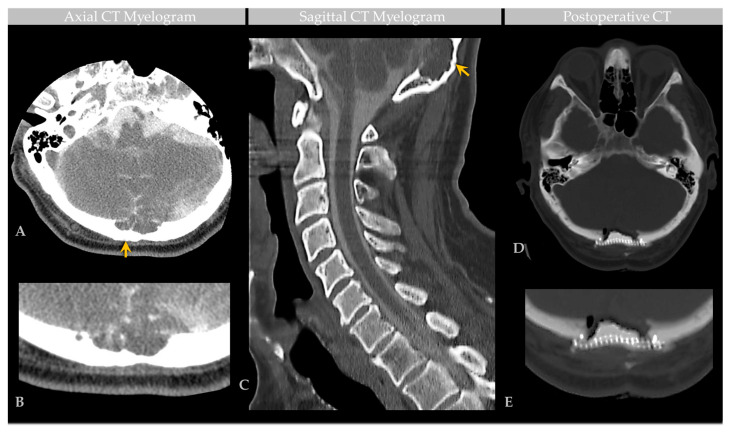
Case 2: CT Myelogram of an individual with GAG involving the confluence of sinuses. Axial sections of CT myelogram, with zoomed image, show contrast partly filling the GAG, as shown on two contiguous levels (A,B). Sagittal image (C) shows soft tissue attenuation within the GAG with defect of the inner table, diploic expansion, and endosteal scalloping of the outer calvarial cortex (arrow). Postoperative CT (D) demonstrates occipital cranioplasty with bone cement and metallic mesh following lesion resection, as shown to advantage on zoomed section (E). Images are from the same individual depicted in Figure 2.
